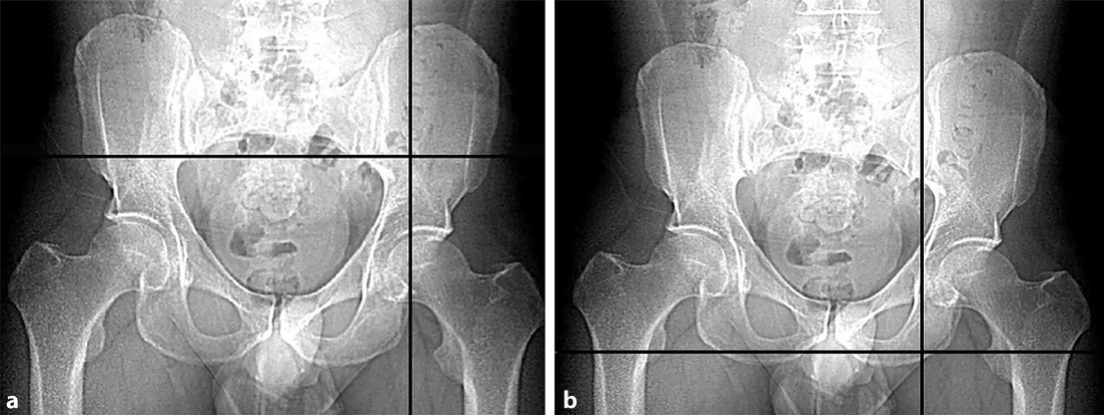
Estimation of the starting point and endpoint of the ideal screw trajectory. This is one of the major challenges in fluoroscopically controlled posterior lag screw fixation. The preoperative planning is performed using multiplanar CT reformations in all three dimensions. The intraoperative application, however, is performed using two-dimensional (2D) fluoroscopic control. The CT scout image is used to facilitate this transition. It is a low-dose pelvic X‑ray routinely obtained during standard CT imaging, which is usually performed in a standard supine position and thus corresponds to intraoperative positioning of the patient. Pads or pillows supporting the lumbosacral region modify the pelvic tilt and should therefore not be used intraoperatively. Software tools allow for a real-time localization of any arbitrary CT point in the scout view (“LiveSync” feature). Thus, the surgeon is able to transfer the ideal screw starting (a) and endpoint (b) identified in multiplanar CT reformations to the 2D anterior–posterior (ap) view scout image. This greatly facilitates identification of the ideal localization of the screw starting and end point (and therefore of the screw trajectory) in the intraoperative pelvic ap view
